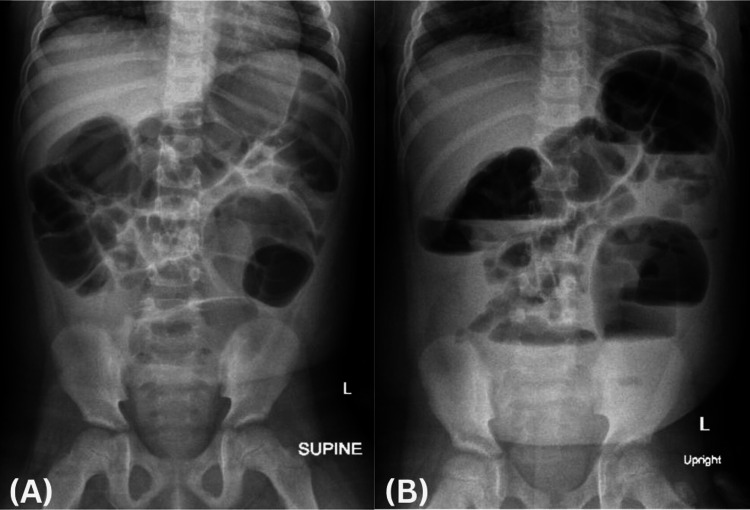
Initial radiographic evaluation at presentation to the emergency department. Anteroposterior abdominal radiographs obtained in the (A) supine and (B) upright positions demonstrate diffuse colonic distention with maximal diameter of 6 cm and no rectal air.
